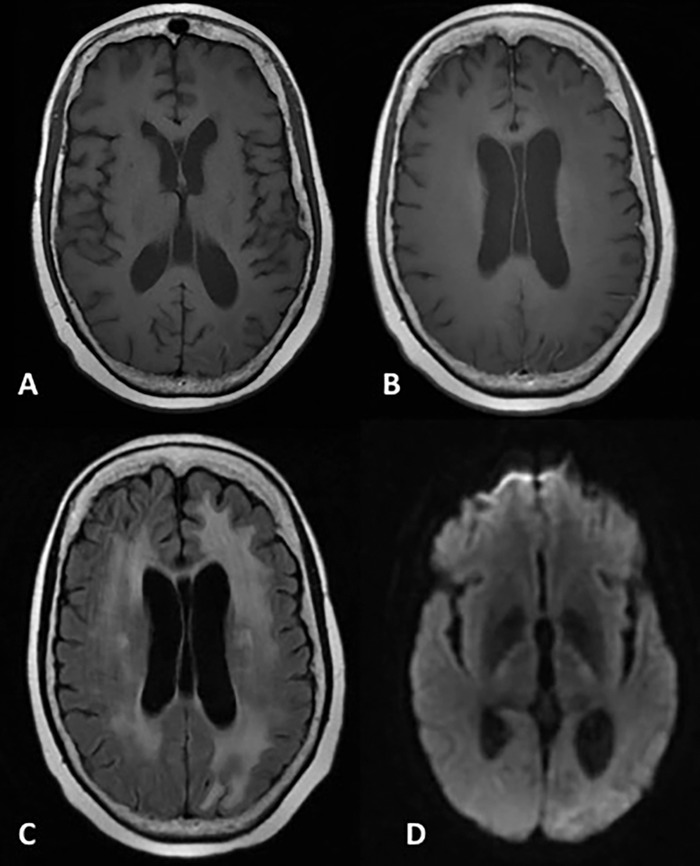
Magnetic resonance imaging at 5-month follow-up. Axial T1-weighted images before (A) and after (B) the administration of gadolinium contrast demonstrate minimal cortical intrinsic T1 shortening along the left posterior parietal and occipital cortex consistent with laminar necrosis but complete resolution of the postcontrast cortical enhancement. T2/fluid attenuated inversion recovery image demonstrates resolution of the posterior cerebral cortical signal abnormality but new left occipital subcortical hyperintensity, indicative of gliosis and encephalomalacia (C). Diffusion weighted imaging shows resolution of diffusion restriction (D).
